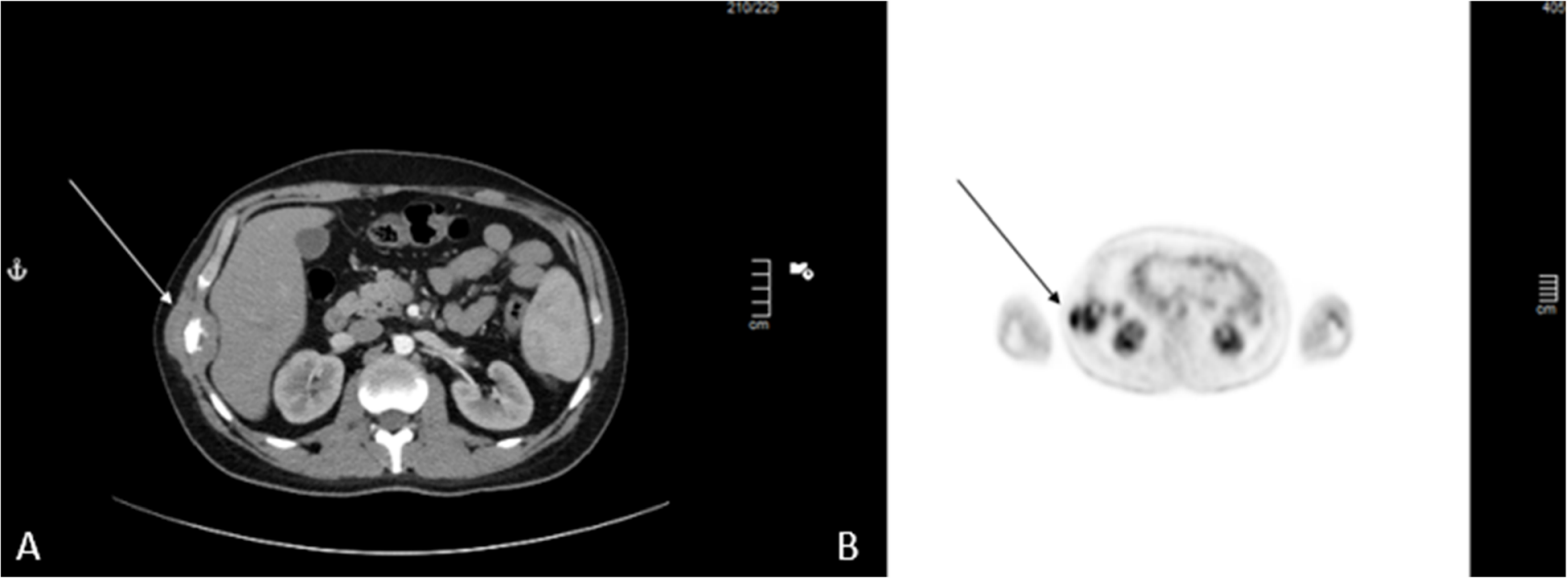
Preoperative CT and PET scans showing tumor location
